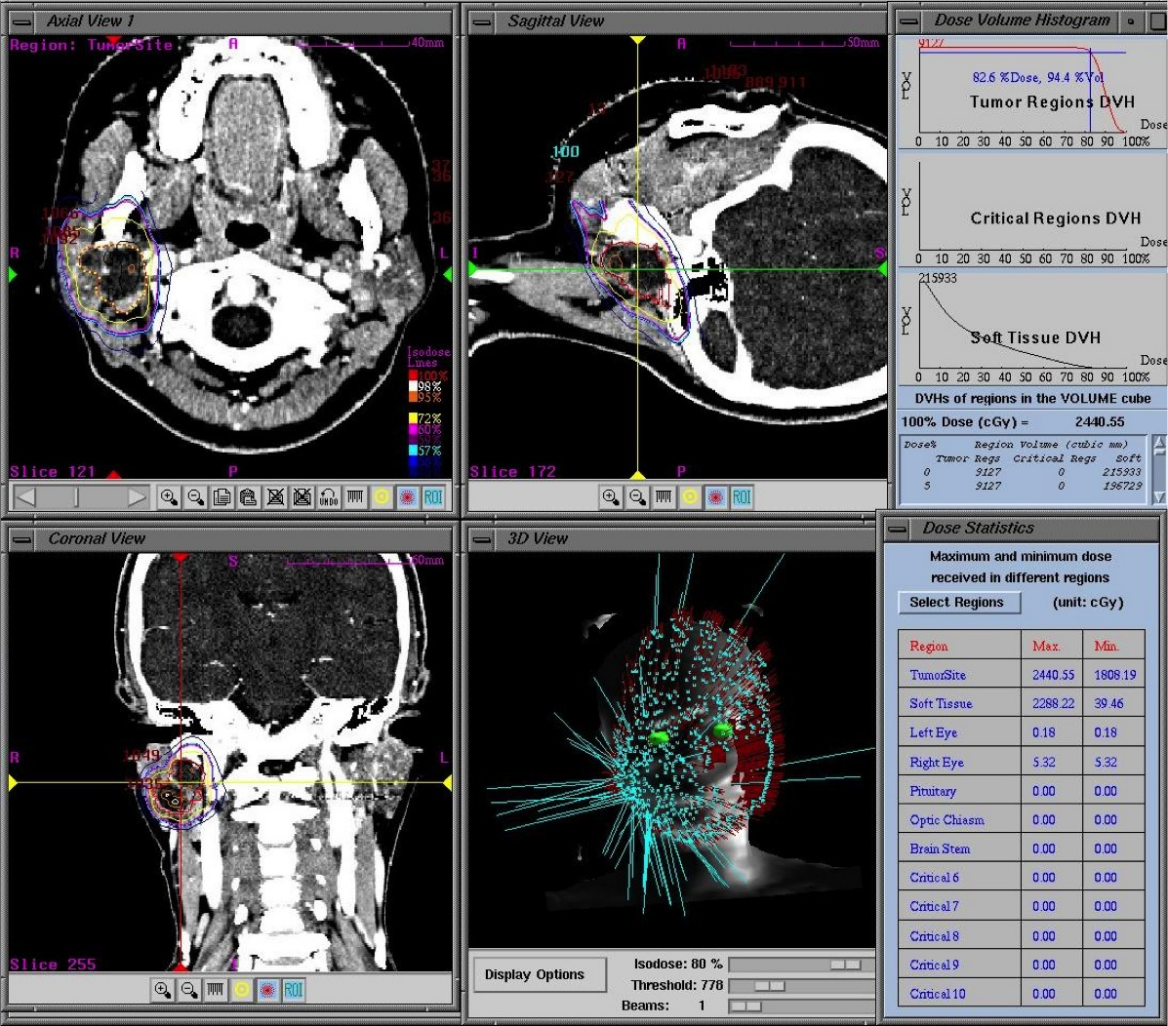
CyberKnife planning images obtained from contrast-enhanced CT images.
